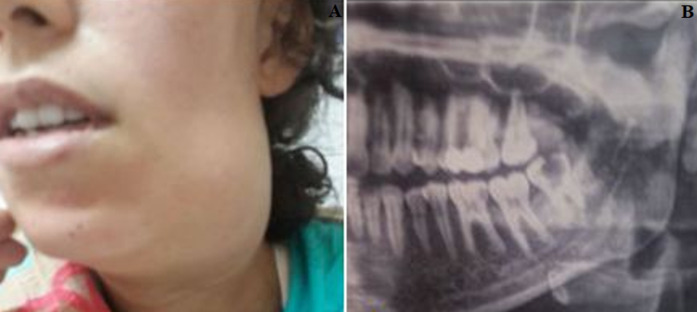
A) picture shoswing extensive submandibular space infection resulting in facial swelling and limited mouth opening; B) orthopantomogram of the same patient showing a carious third molar (N°38)
